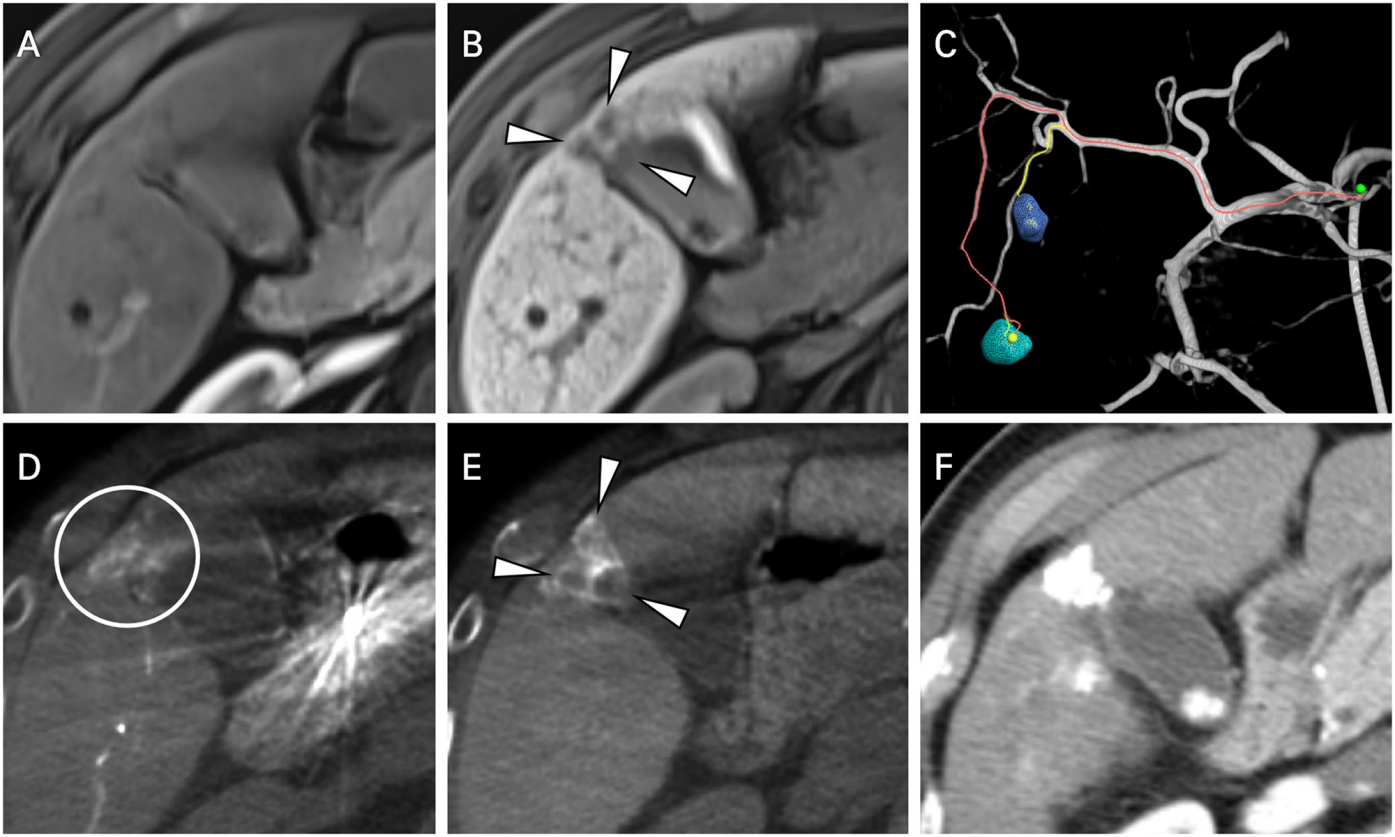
A 64-year-old male patient with recurred HCCs in segments 5 and 6 (Child–Pugh class A, BCLC stage B). (A) In the AP of MRI, there was no definite enhancing lesions at the gallbladder bed of the liver. (B) Three nodular hypodense lesions were identified in the hepatobiliary phase. These lesions were concluded to be indeterminate nodules upon reading (white arrowheads). (C) A preexisting tumor in segment 6 and its feeder are visualized with a yellow line by the EmboGuide^®^ program. An additional tumor (found by DP-CBCT) in segment 5 and its feeder are visualized with a red line. The treatment plan was changed during the procedure, with additional chemoembolization performed on segment 5. (D) Faint enhancement was visualized on AP-CBCT with an unknown degree of clinical significance (white circle). (E) Three rim-enhancing lesions were detected on delayed-phase CBCT (white arrowheads). (F) On three-month follow-up CT imaging, compact lipiodol uptake without residual arterial enhancement was visualized after TACE.
